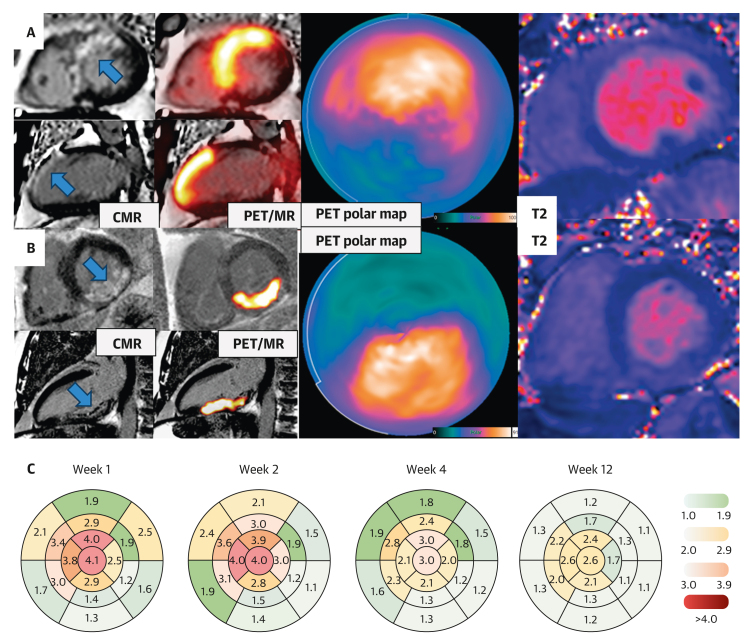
Regional Fibroblast Activation After Acute Myocardial Infarction (A, B) Cases of acute myocardial infarction (imaged 23 days (A) and 3 days (B) after myocardial infarction) affecting the anterior (A) and inferior (B) walls. Short-axis and 2-chamber magnetic resonance views are shown fused with PET. Late gadolinium enhancement is evident in the anterior (A) and inferior (B) walls, respectively (blue arrows). Intense [^68^Ga]FAPI-46 activity (TBR_max_ = 3.80 [A], 6.30 [B]) is observed in the infarct zone but extends into the peri-infarct region. Matched polar maps demonstrate increased anterior and inferior [^68^Ga]FAPI-46 signals, respectively, and are presented alongside the matched T2-weighted short-axis images. (C) TBR_max_ within each myocardial segment for the acute anterior myocardial infarcts are presented across time. Emboldened values seen in segments 7-9, 13-15, and 17 demonstrate the myocardial segments with a statistically significant change over time (*P* < 0.05). [^68^Ga]FAPI-46 = ^68^Gallium-fibroblast activation protein inhibitor-46; CMR = cardiac magnetic resonance; PET = positron emission tomography; TBR_max_ = maximal target-to-background ratio.
